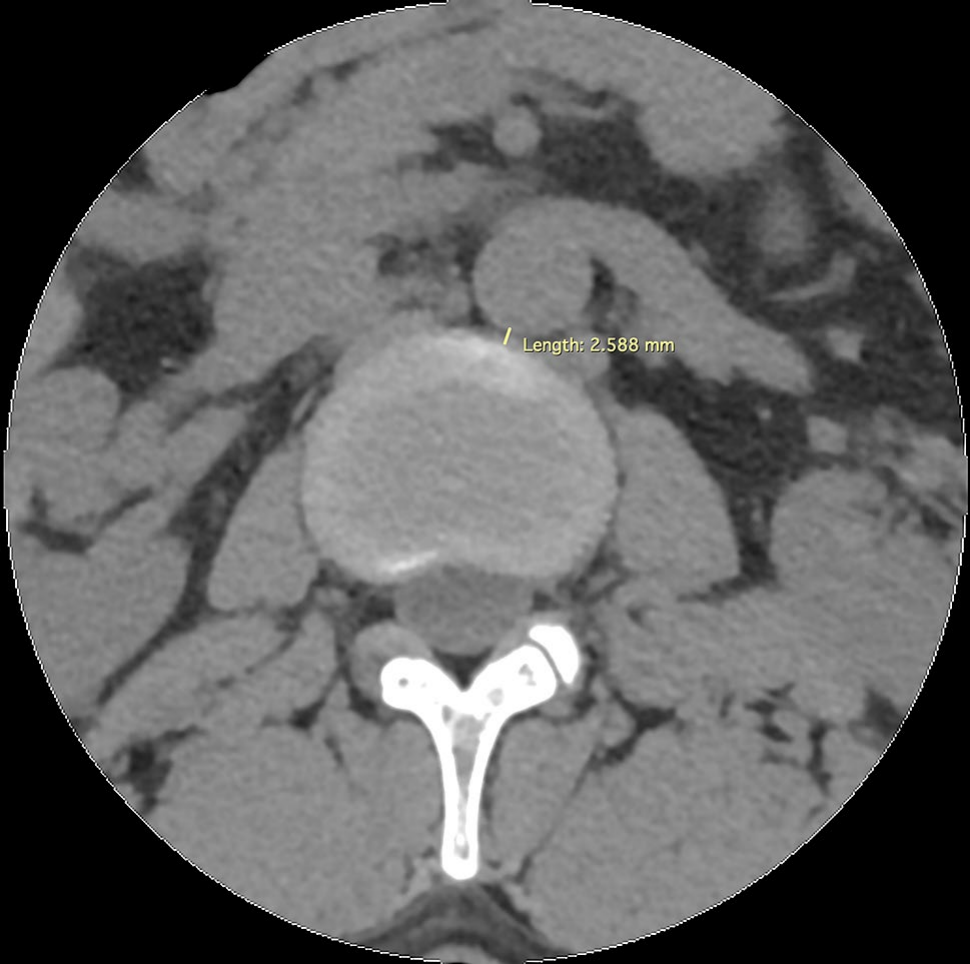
Lumbar spine computed tomography (axial) measurement of arterial-disc distance
